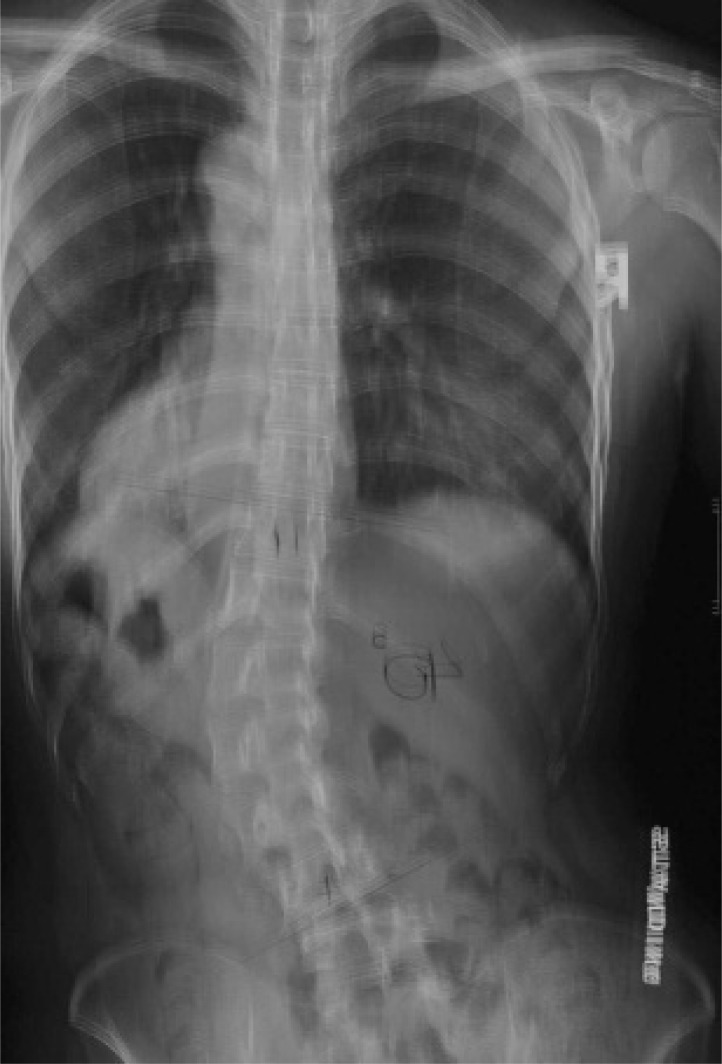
Rotation of vertebral body and spinous process in scoliosis. From the x-ray, it is apparent that the vertebral body of L1 (the apex of the curve) rotates towards the convexity of the curve, which is the left and the spinous process rotates to the concavity of the curve.
